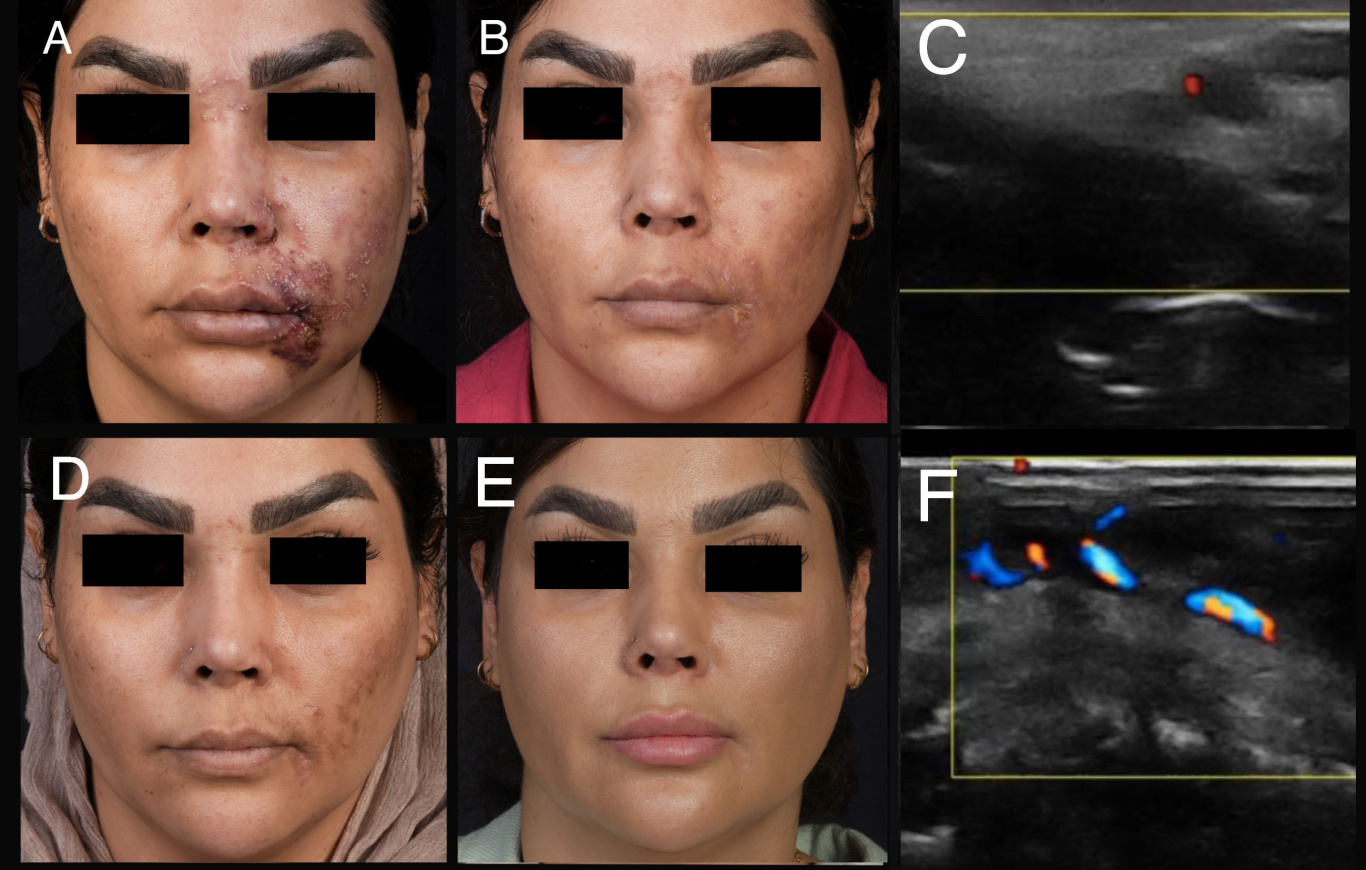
A 40-year-old female experienced stage three of ischemia following an injection of 1 cc HA filler. (A) A clinical presentation after 74 h of filler injection. (B) Improvement of ischemia after 2 weeks. (C) An ultrasound examination before treatment (Involvement of facial artery). (D) Improvement of ischemia a month after filler injection. (E) Complete improvement 3 months after “THIS and FAT” protocol. (F) An ultrasound examination after treatment (arterial flow return to normal).
